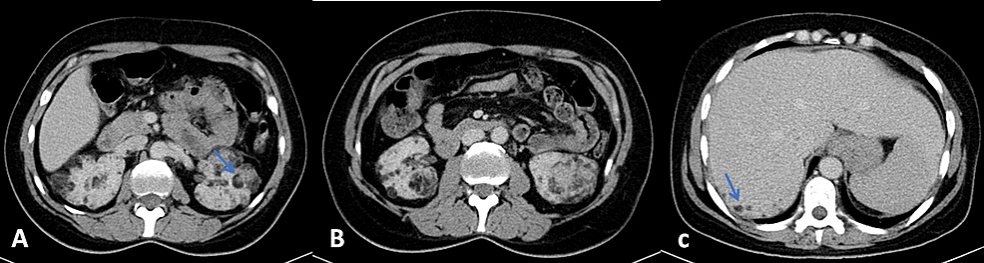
Abdominopelvic CT. Multiple nodules in the parenchyma of both kidneys compatible with angiomyolipomas (A and B, blue arrow). In the liver parenchyma, some nodular formations (C, blue arrow) corresponding to intrahepatic angiomyolipomas can be seen. CT: computed tomography
